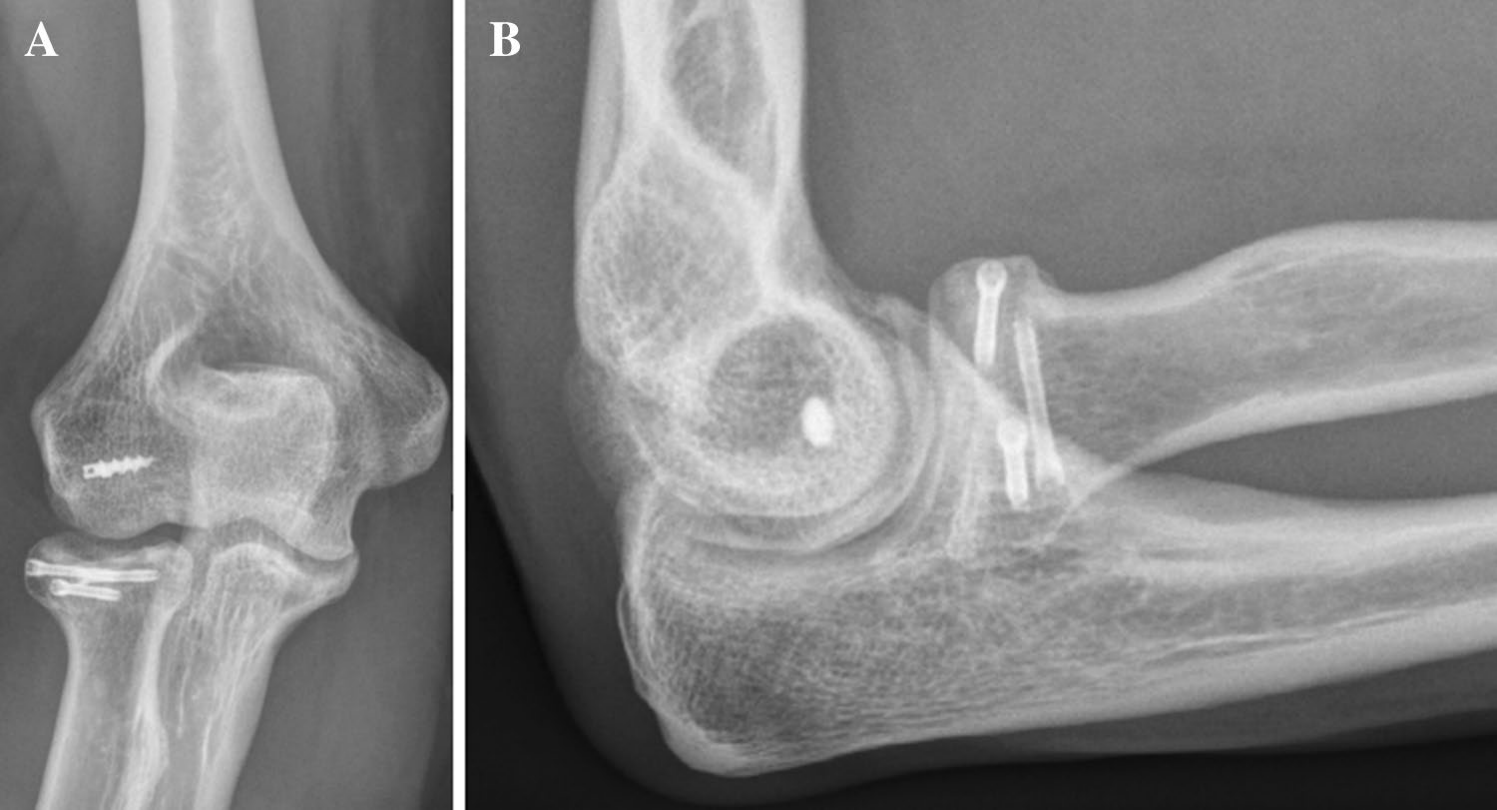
a, b Postoperative radiographs (anteroposterior and lateral) after radial head fixation with three CCS and reattached LCLC after 6 months (Case 1)
